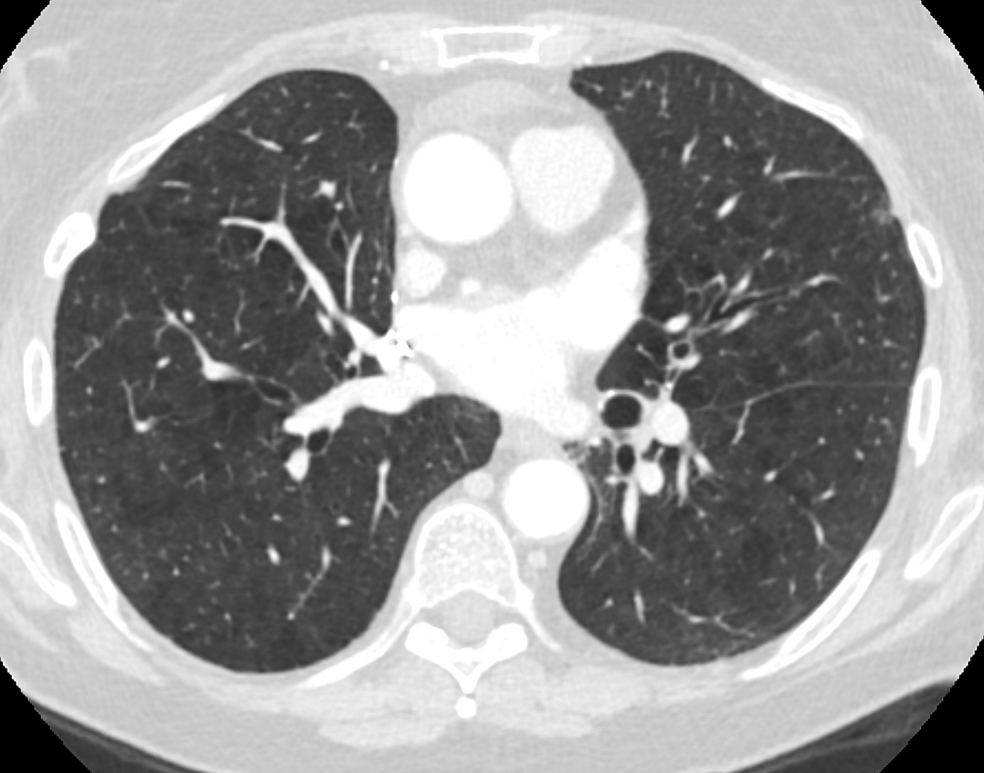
Chest CT with intravenous contrast done two months prior to the current admission revealed no airspace abnormalities.
